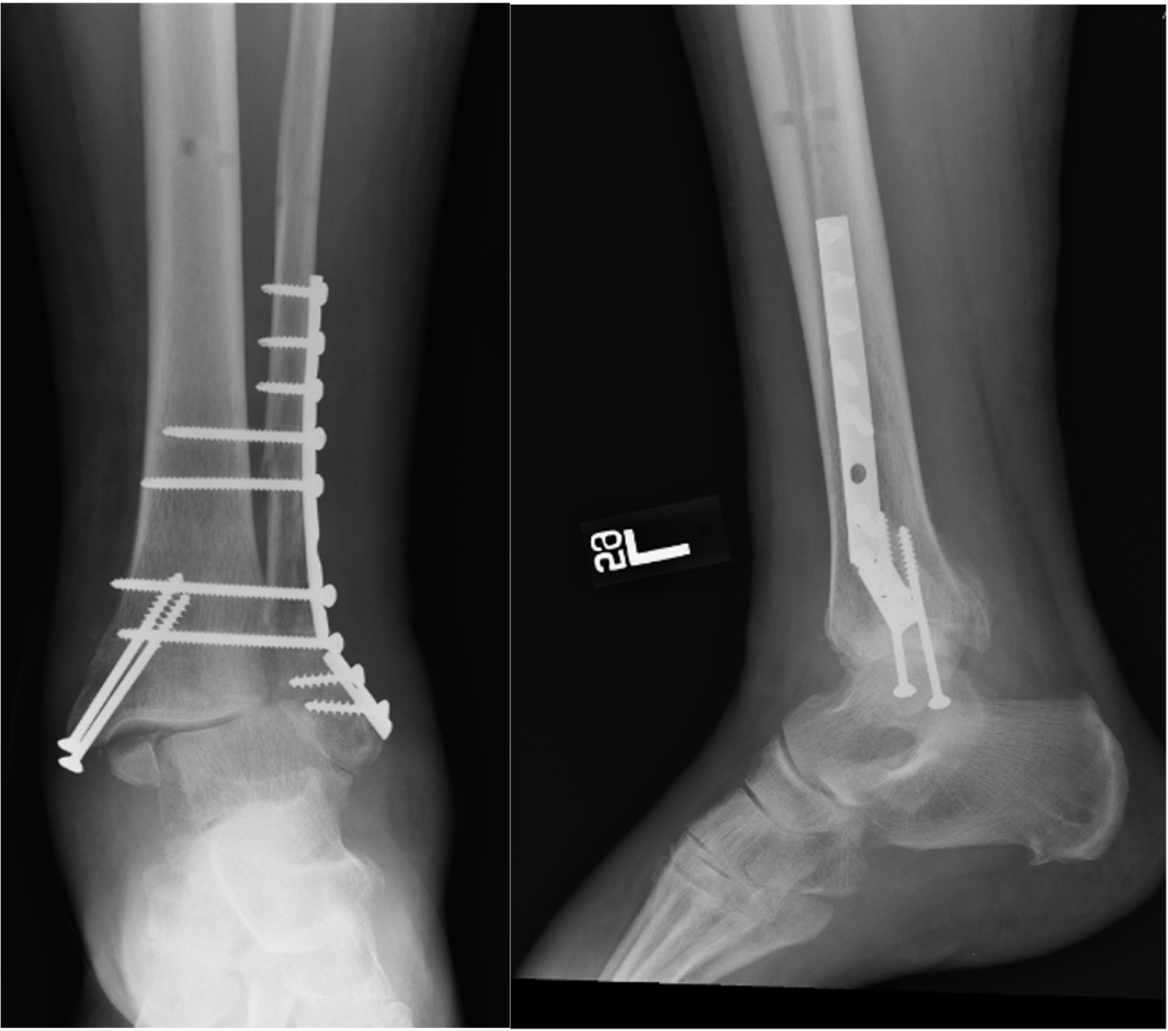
Postoperative radiographs demonstrating open reduction and internal fixation (ORIF) for an unstable ankle fracture. Standard anteroposterior and lateral views show anatomic restoration of the tibiotalar joint with plate-and-screw fixation of the fibula and supplemental fixation of associated medial or posterior malleolar fragments.
